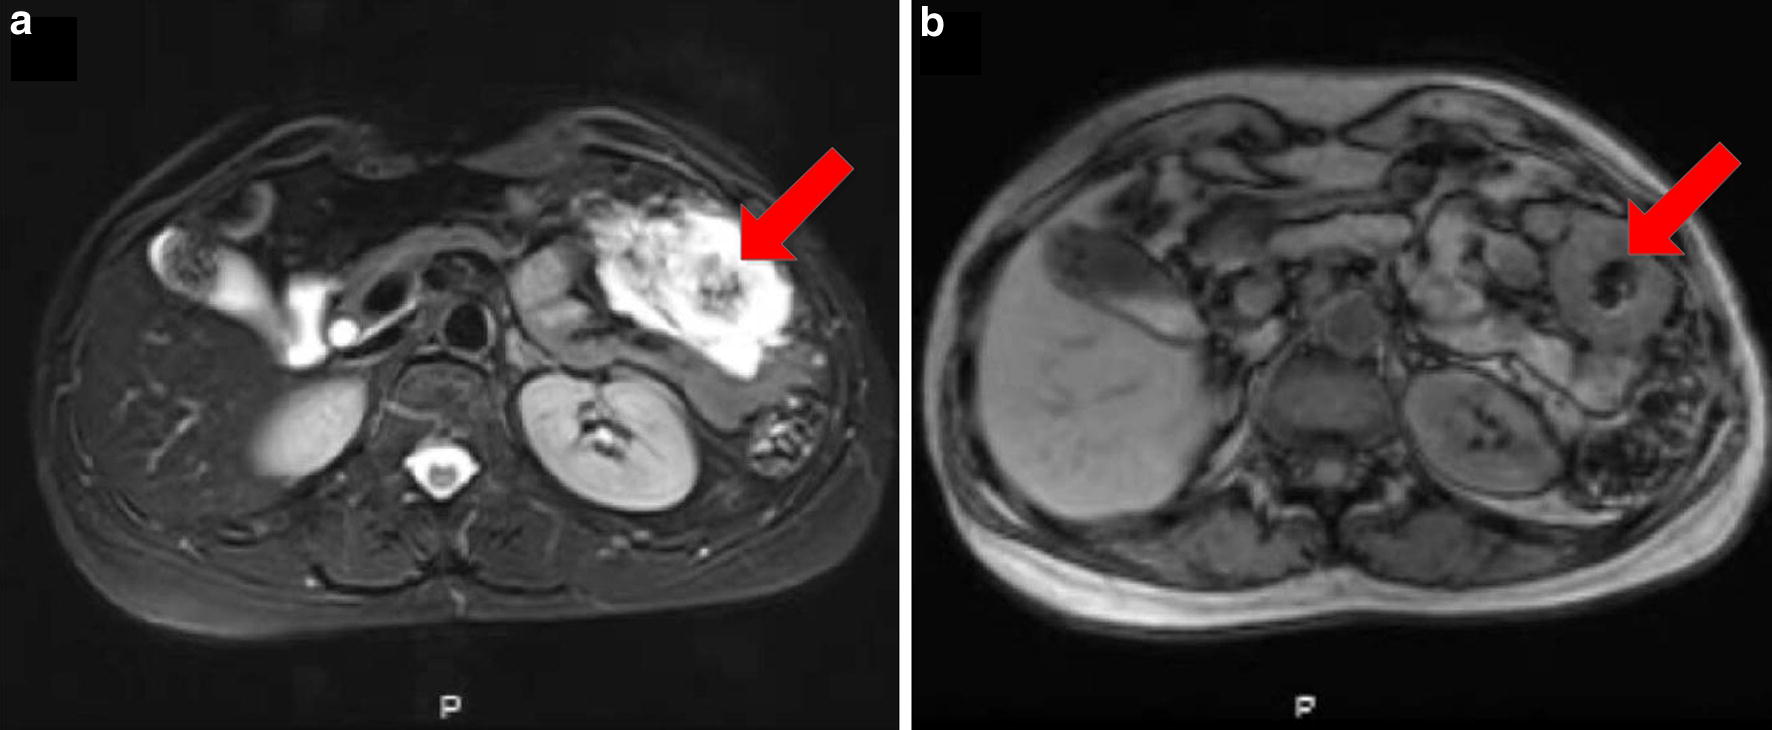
Magnetic resonance images showed an ulcerative mucinous colorectal adenocarcinoma (5.5 × 3.0 × 2.5 cm) located in the transverse colon 55 cm from the anus in a 53-year-old female patient. The patient was diagnosed as mucinous colorectal adenocarcinoma staged T4N2M1 with liver and abdominal metastases. She received 3 cycles of XELOX (capecitabine plus oxaliplatin) chemotherapy, a palliative surgery, 5 cycles of XELOX chemotherapy and capecitabine maintenance therapy for 5 months till present. a The axial T2-weighted imaging showed a significantly more intense signal on mucin pools (red arrow) than normal muscle. b In the axial T1-weighted image, the mucinous component (red arrow) showed similar signal intensity as to normal muscle
